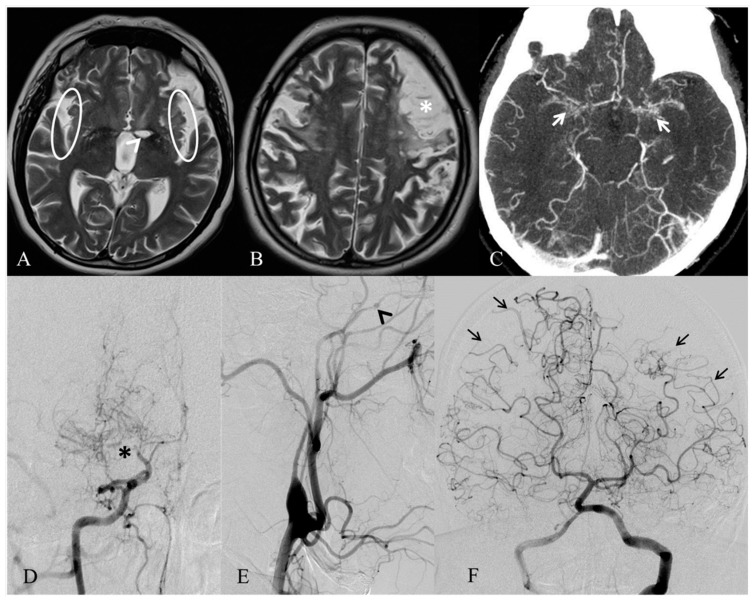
MRI and angiographic images of MA of our case. Axial T2-weighted MRI (A,B) exhibits only a few vascular voids in the Sylvian fissures (white circles), due to a reduced flow in both the middle cerebral arteries. A well-demarcated ischemic stroke in the left nucleo-capsular region (white arrowhead) and an infarct involving the left frontal lobe (white asterisk) are also noticeable. Axial angio-CT (C) shows proliferation and enlargement of the lenticulostriate arteries (white arrows) in the basal ganglia. Right and left common carotid arteries’ angiograms (D–E) demonstrate a distal stenosis of the right internal carotid artery (black asterisk), with development of prominent collateral vessels, giving the characteristic puff of smoke appearance of moyamoya disease, and an occlusion of the left internal carotid artery, distally to the ophthalmic artery (black arrowhead). Left vertebral artery angiogram (F) indicates leptomeningeal cortical anastomosis from both the posterior cerebral arteries to the parietal and temporal lobes (black arrows).
